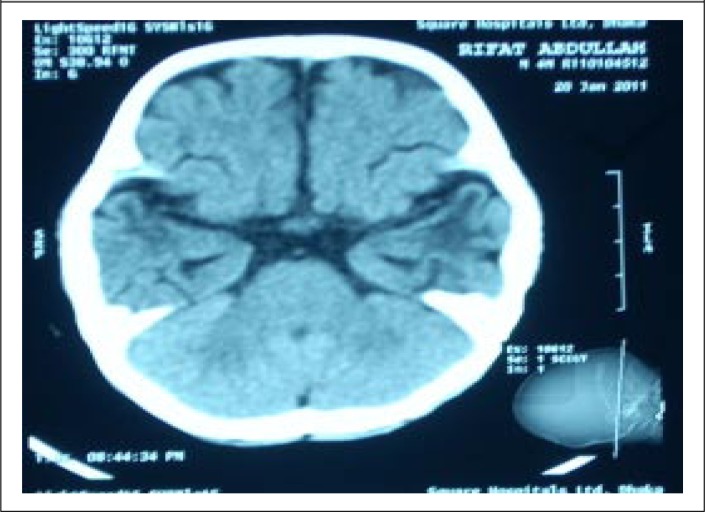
CT scan: Bilateral low-attenuation areas are seen in both temporal poles, overlying cortex is spared
